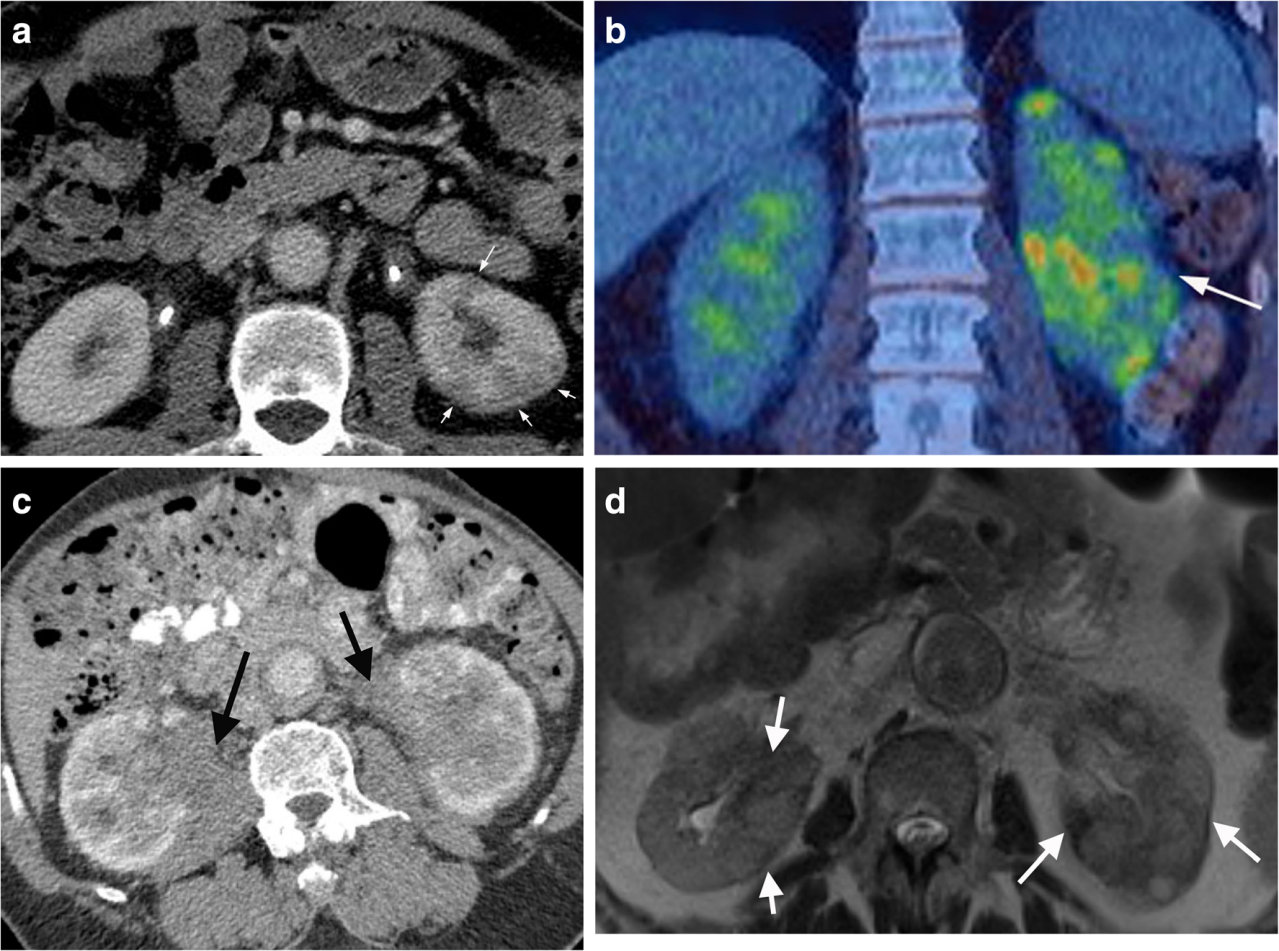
Example of IgG4 disease affecting the left kidney. a Axial CECT shows typical wedge-shaped low-density renal cortical lesions in the left kidney (*arrows*). Both kidneys are stented as there was also IgG4 related retroperitoneal fibrosis. b Coronal FDG PET/CT in same patient as in a showing multifocal high-grade uptake in the left kidney (*arrow*). c Example of bilateral renal pelvic involvement in a different patient (*arrows*) on CECT. d Axial T2 turbospin echo image in different patient shows renal lesions (*arrows*) are of mixed high and low T2 signal (typically reported in the literature as low T2 signal)
